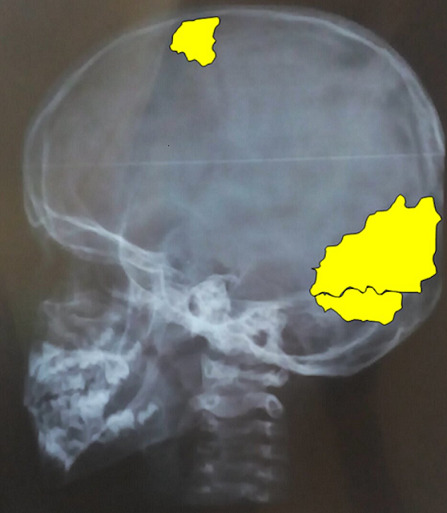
skull radiograph showing Wormian bones; note the frontal bossing and the small upper and lower jaw
